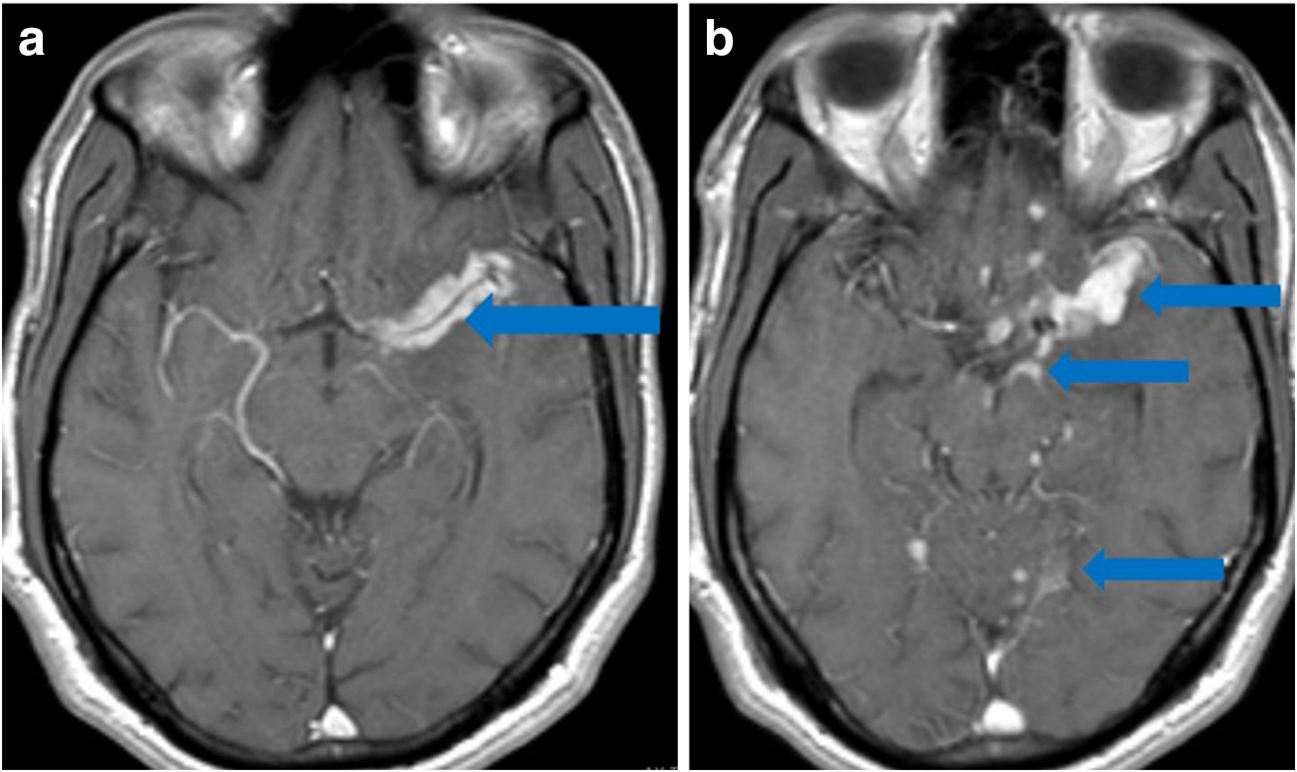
Leptomeningeal enhancement and encephalitis in a 39-year-old with neurologic impairment: a Axial post-contrast MRI demonstrates leptomeningeal enhancement along the left middle cerebral artery (MCA) distribution (blue arrow). b Axial post-contrast MRI demonstrates leptomeningeal enhancement along the left MCA, as well as the basal cisterns and superior cerebellum (blue arrows).
